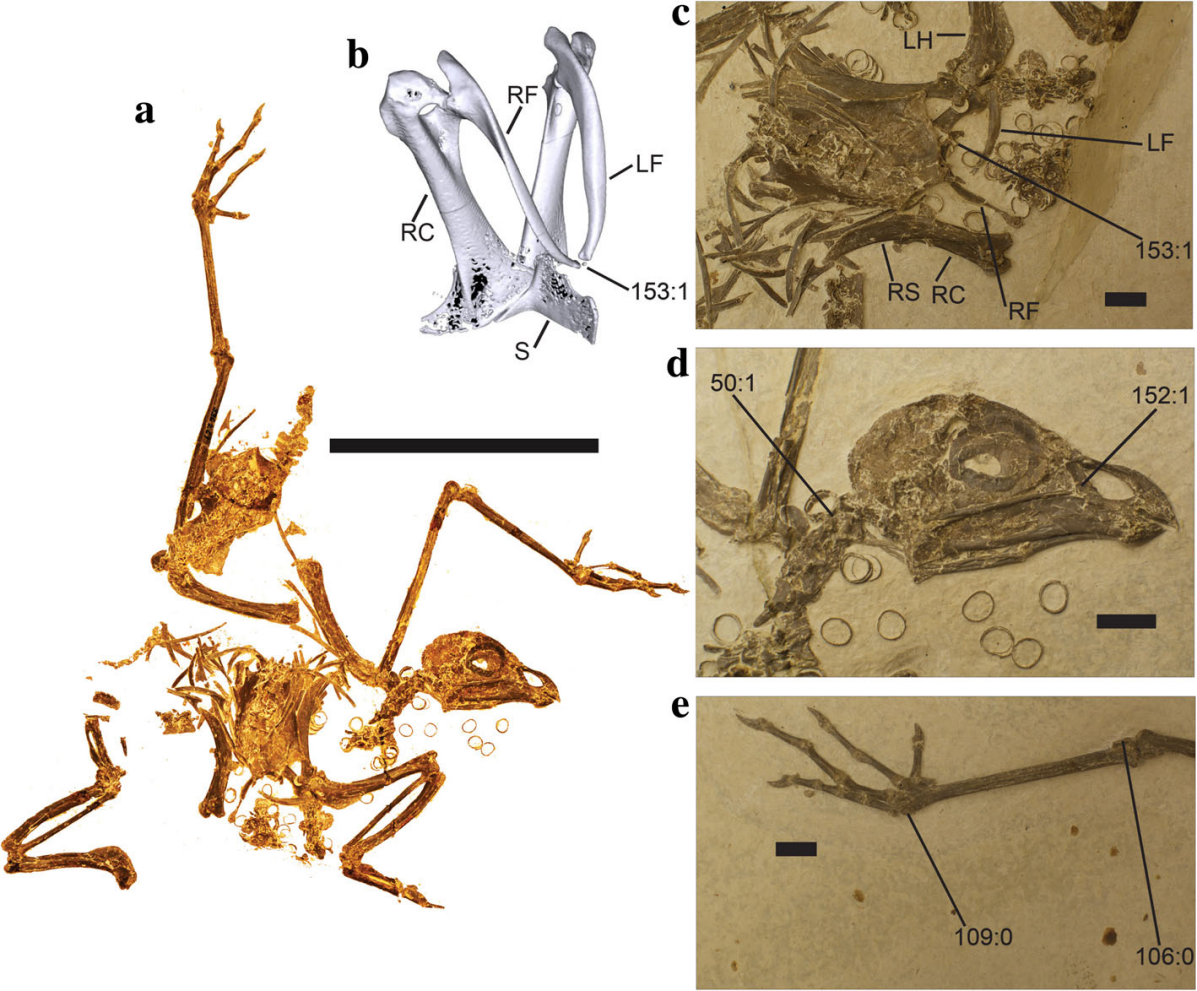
Skeletal morphology of total clade musophagids. (*a*) Complete skeleton of *Foro panarium* holotype USNM 336261. Scale bar equals 10 cm. (*b*) 3-dimensional CT rendering of the pectoral region of Ross’s Turaco (*Musophaga rossae*) GCO 1142 (Georgia College Ornithology, Georgia College and State University, Milledgeville, GA). LF – left ramus of furcula, RC – right coracoid, RF – right ramus of furcula, S – sternum. 153:1 denotes unfused midline of furcula, which optimizes as an unambiguous synapomorphy of a *Foro* + Musophagidae clade. (*c*) Pectoral region of *F*. *panarium*. LH – left humerus, RS – right scapula. (*d*) Cranial region of USNM 336261. 50:1 processus costales of axis absent. 152:1 bill short and stout with broad processus maxillaris of the os nasale. (*e*) Distal end of right leg of USNM 336261. 109:0 – trochlea metatarsi IV without large trochlea accessoria. 106:0 tendon of musculus flexor hallucis longus not enclosed in bony canal
